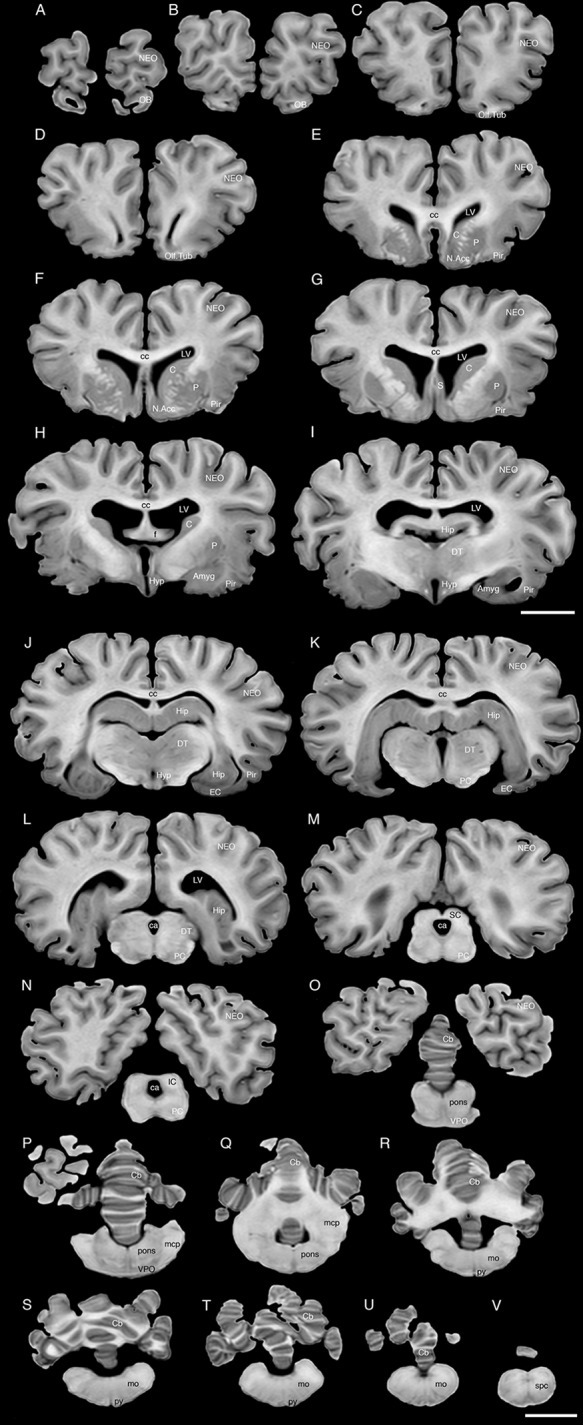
A series of coronal structural MR images through the brain of the black rhinoceros (*D. bicornis*). (A) is the most rostral section and (V) is the most caudal section, with each section having a thickness of 2 mm and each section being 6 mm apart. Note the typically mammalian topography of the various regions and structures of the brain. Scale bar = 2 cm. Amyg, amygdaloid body; C, caudate nucleus; ca, cerebral aqueduct; Cb, cerebellum; cc, corpus callosum; DT, dorsal thalamus; EC, entorhinal cortex; f, fornix; Hip, hippocampus; Hyp, hypothalamus; IC, inferior colliculus; LV, lateral ventricle; N.Acc, nucleus accumbens; NEO, neocortex; mcp, middle cerebellar peduncle; mo, medulla oblongata; OB, olfactory bulb; Olf. Tub, olfactory tubercle; P, putamen; PC, cerebral peduncle; Pir, piriform cortex; py, pyramidal tract; S, septal nuclear complex; SC, superior colliculus; spc, cervical spinal cord; VPO, ventral pontine nucleus.
